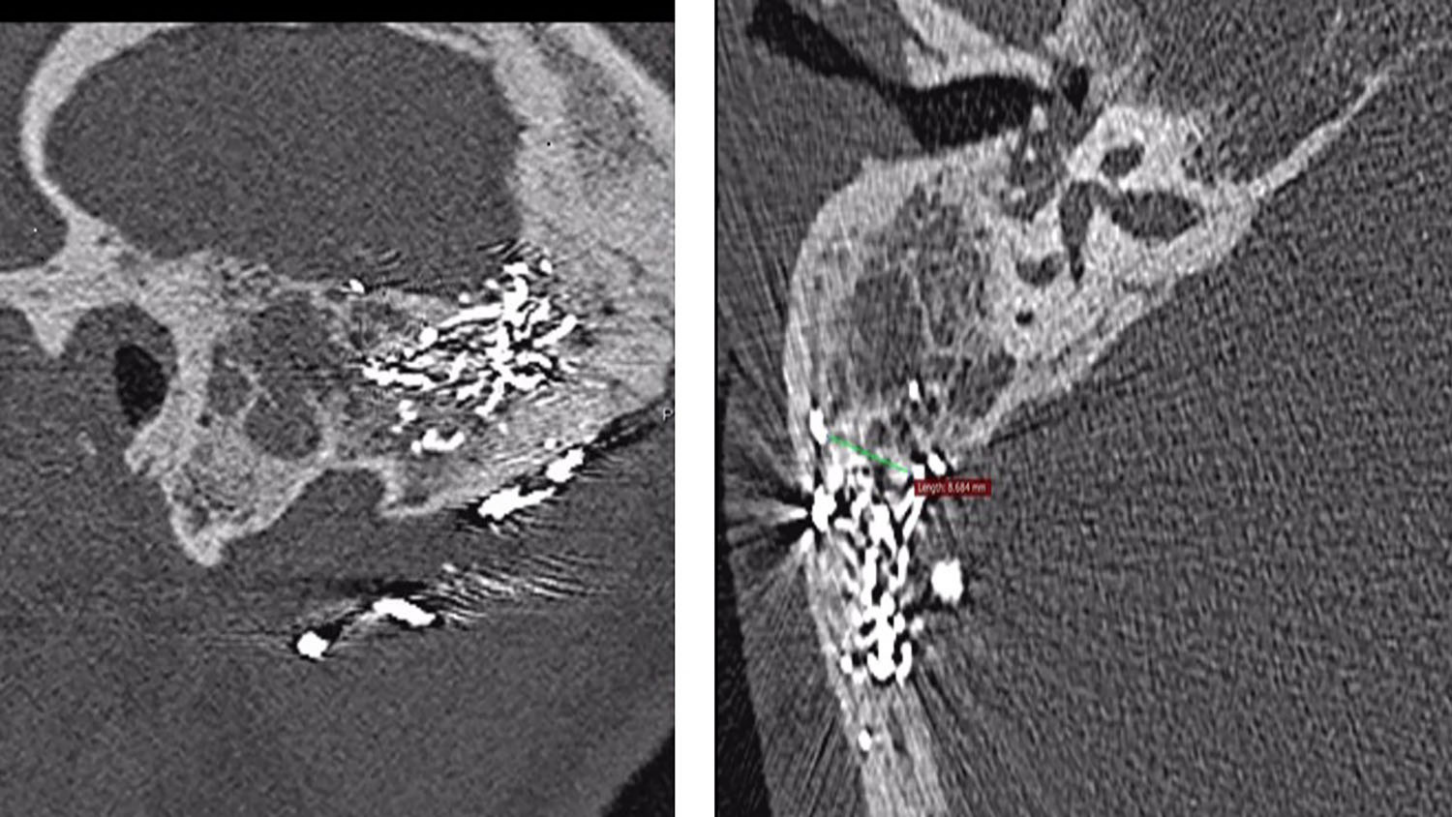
High-resolution CT axial and sagittal imaging of the temporal bones: Onyx in the temporal bone and in the extracranial venous circulation
